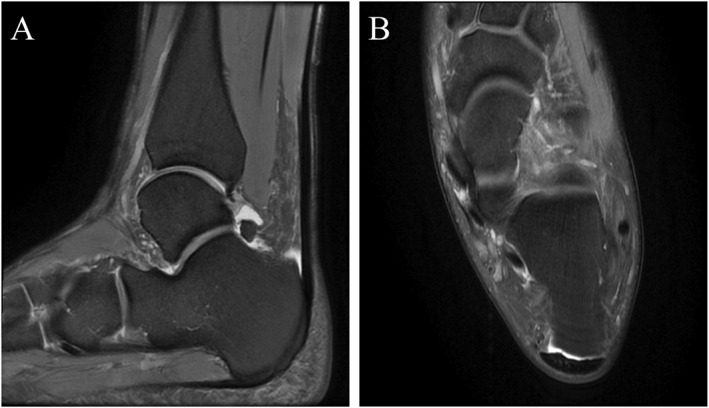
A 31‐year‐old male amateur marathon runner with a 3‐year running experience and a monthly running distance of 120 km; representative MRI of posterior bursitis. Sagittal (A) and axial fs‐PDWI (B) of the left ankle show increased retrocalcaneal bursal effusion (anteroposterior diameter × superoinferior diameter × transverse diameter: 5 × 9 × 12 mm).
